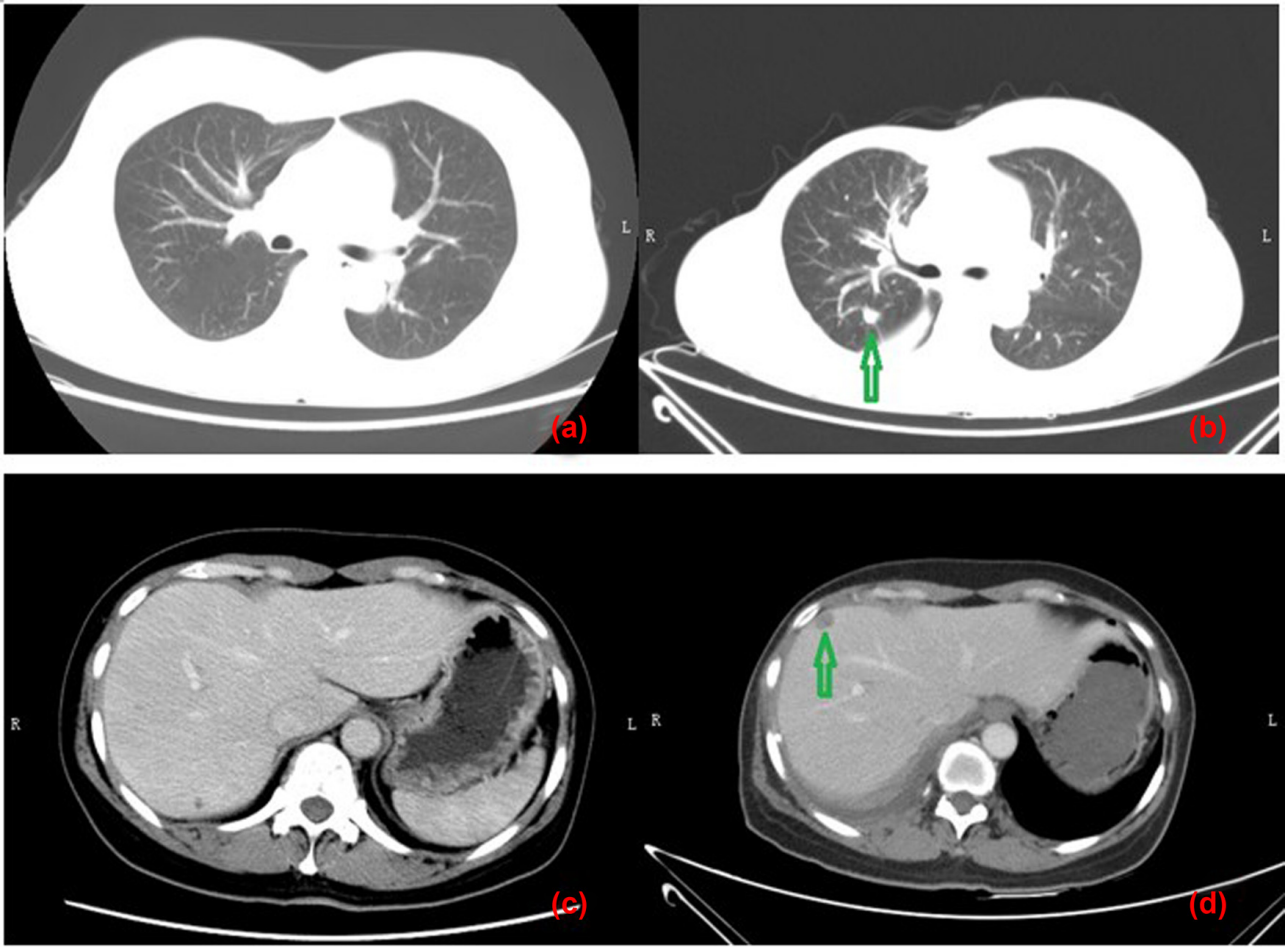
Comparing CT plans of the lung and liver before the modified radical mastectomy and 24 months after the surgery. Images (a) and (c) were CT plans of the lung and liver before the modified radical mastectomy, respectively, and revealed no metastases. Images (b) and (d) were the corresponding plans 24 months after the surgery, and metastases were indicated by the green arrows.
